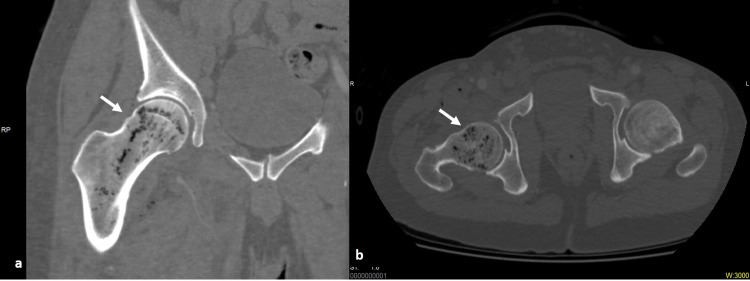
Intraosseous gas locules within the proximal right femur on CT bone window (arrows), coronal (a) and axial (b).
